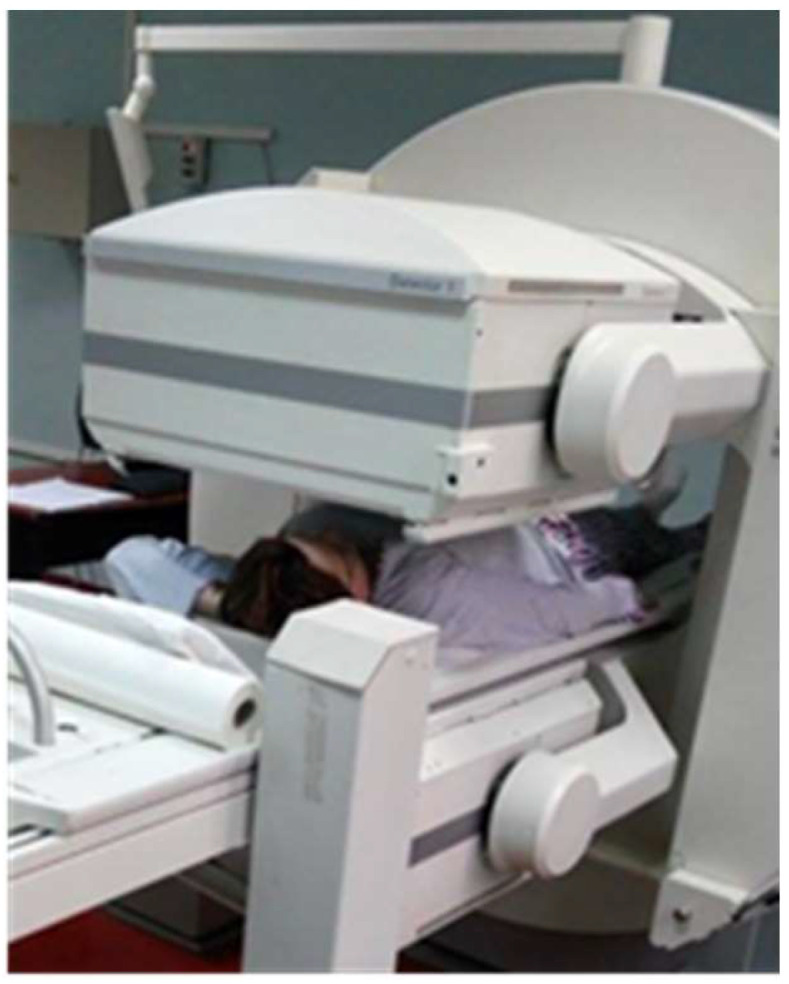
Acquisition of the lymphoscintigraphic image using a classical gamma camera dual detector, Siemens E.CAM from “Sfântul Spiridon” Clinic County Emergency Hospital.
